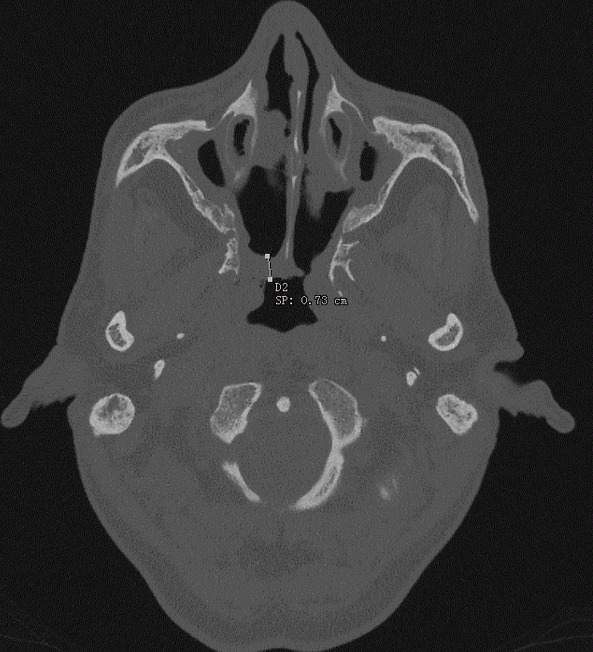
Facial CT scan in the axial section showing a stenosis of both choanae
